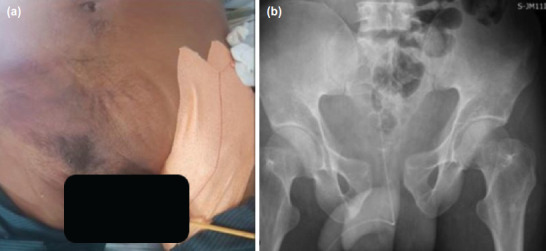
(a) Clinical image showing bruising involving right lower anterior abdominal wall with extension into scrotal sac. (b) Radiograph of pelvis reveals “open-book” pelvic injury with fracture of the left side of the sacrum.
